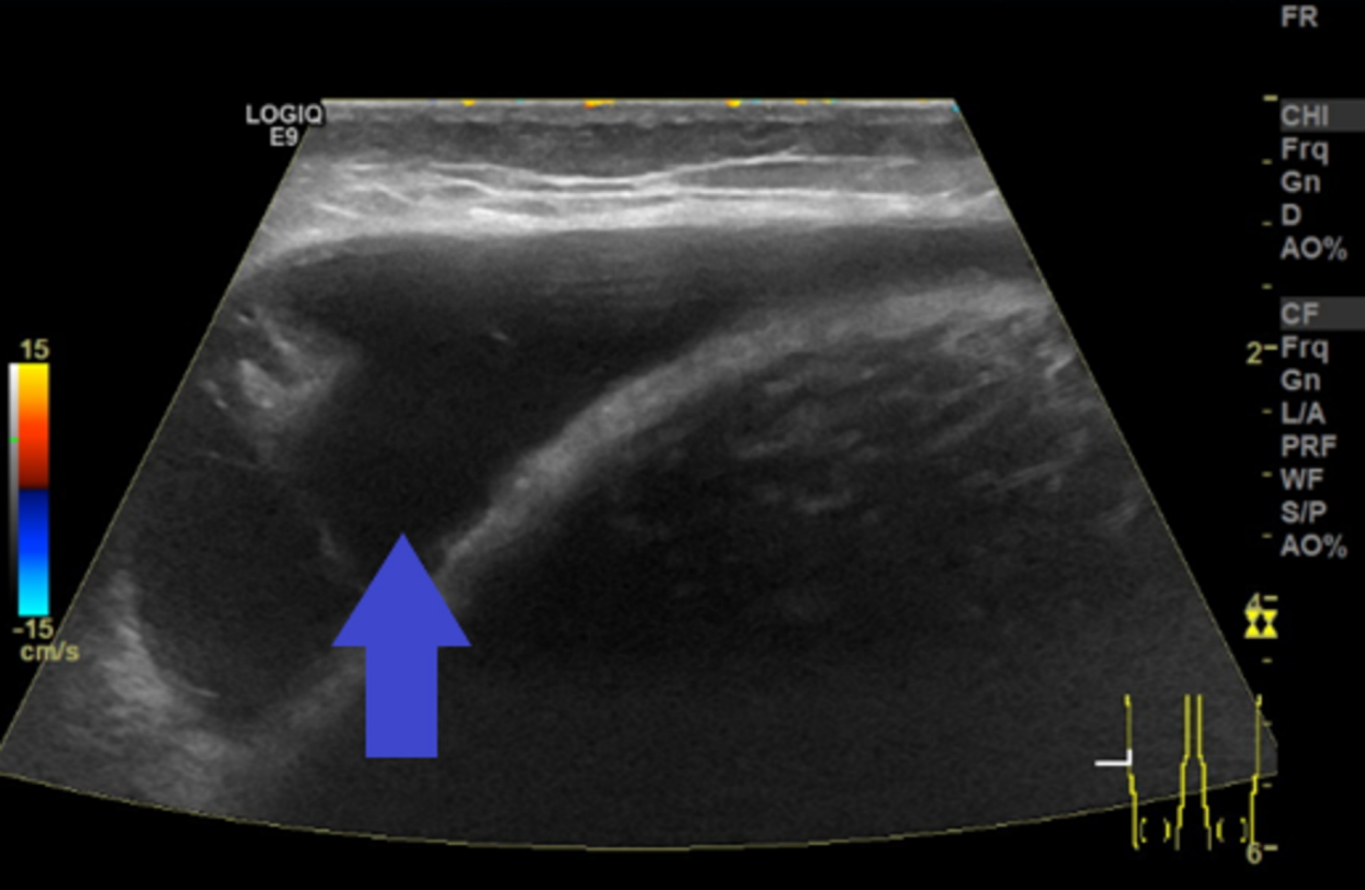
Ultrasound of right lateral thigh showing fluid collection
